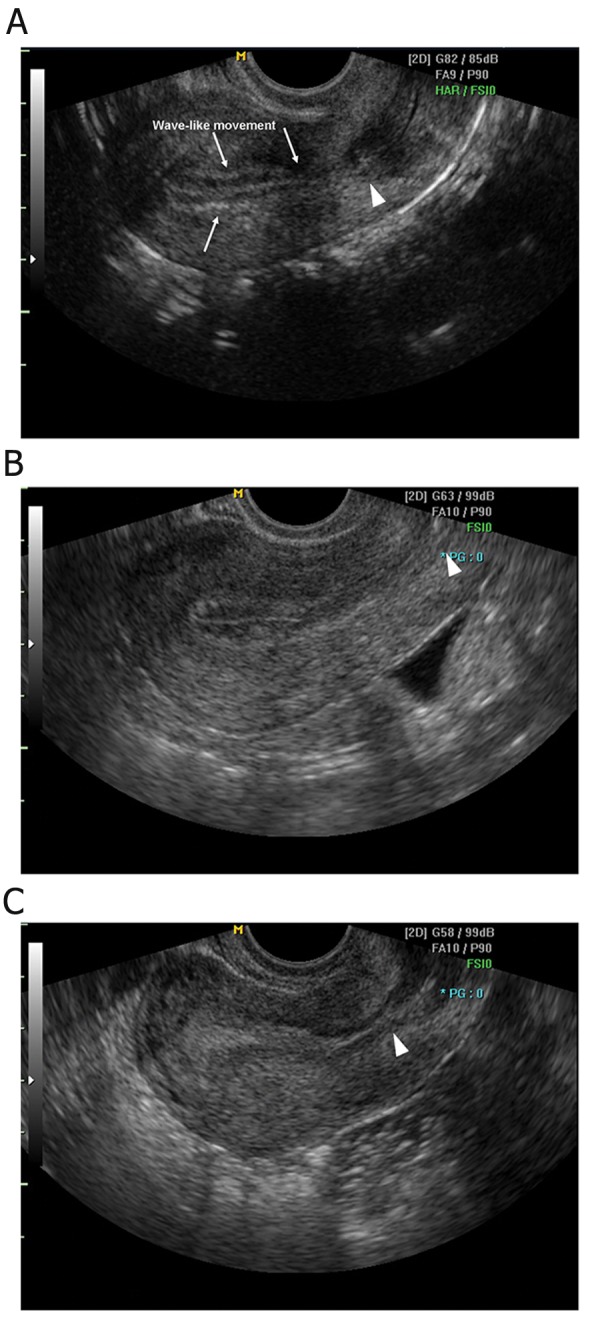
Ultrasound images of endometrium. Echogenisity was decided by the comparison of myomertial echo. Homogeneously hypoechoic endometrium (A) is defined as a marginal hyperechoic line with a prominent hypoechoic inner layer, in which endometrial wave-like movements are observed. Inhomogeneous endometrium (B) shows an endometrium with a hyperechoic margin and a mottled inner layer, and homogeneously hyperechoic endometrium (C) exhibits an inner layer as hyperechoic as a marginal line. Internal os (arrowheads) can be identified.
